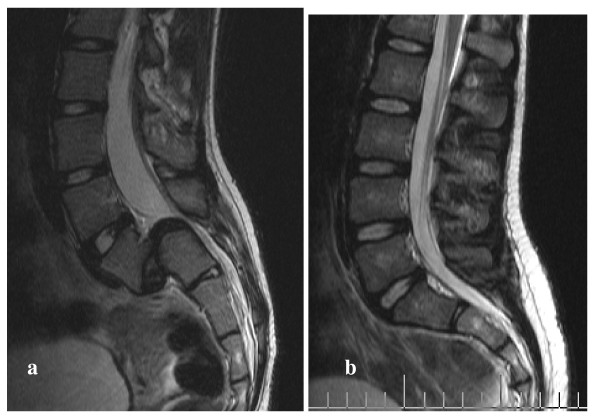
T2-weighted MR images of lumbar spine. T2-weighted sagittal MR images of a patient (female, 13 years old), suffering from spondylolisthesis (a) and a patient (female, 14 years old) suffering from idiopathic scoliosis (b)
